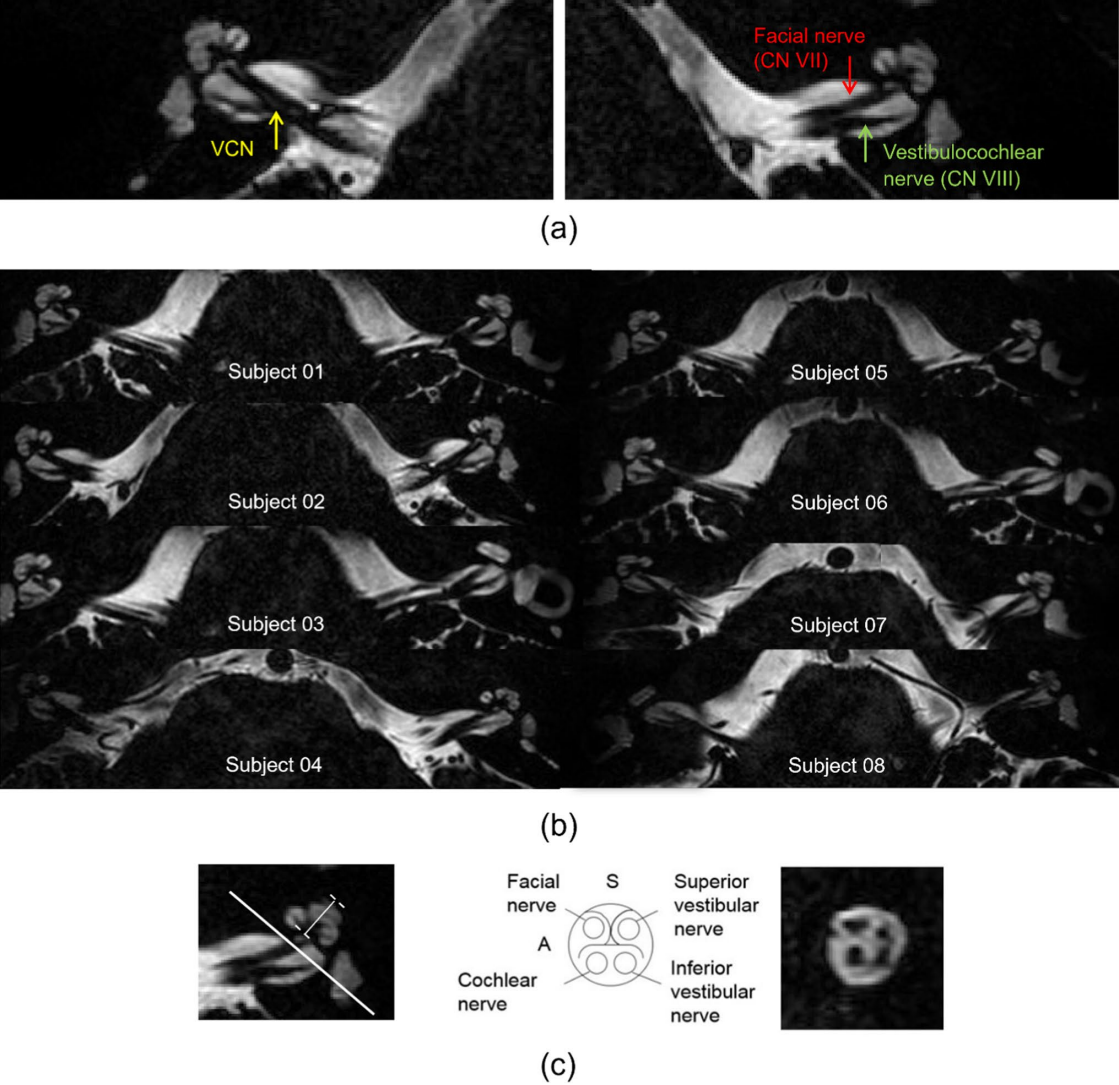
a MR images acquired with dielectric pads, show both sides of the cochlea and the branches of cranial nerves (CN VII, VII). The branching of the vestibulocochlear nerve (VCN) is indicated by the yellow arrow, CN VII by the red arrow, and CN VIII by the green arrow. b The normal anatomy of cranial nerves in the ear canal across eight subjects, which was clearly visible on axial slices at 7 T MRI. c The height measured in the IAC and the cranial nerves (CN VII, VII) on an axial image, running perpendicular to the internal auditory canal
